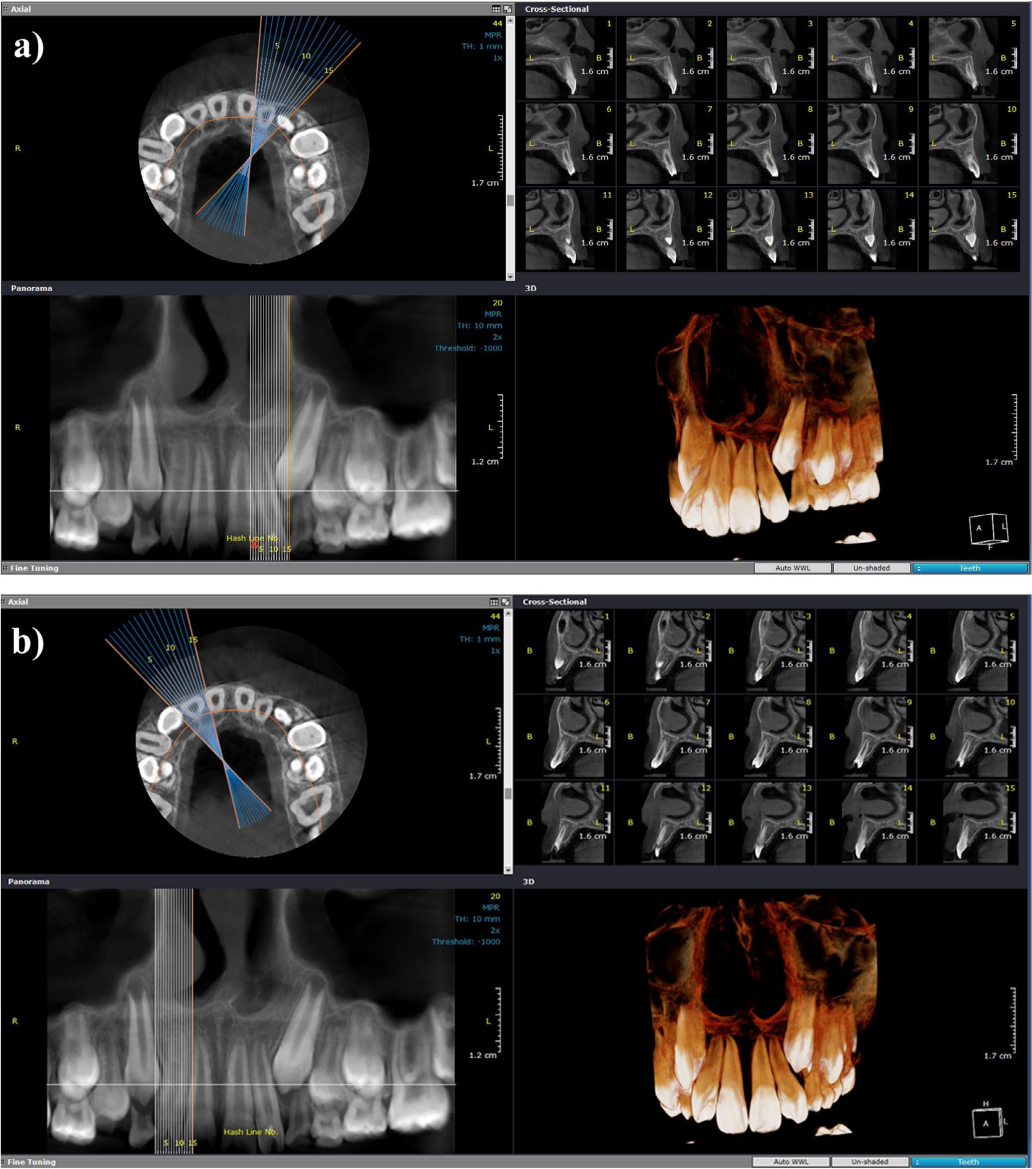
(a, b) Pretreatment computed cone‐beam tomography (CBCT).
